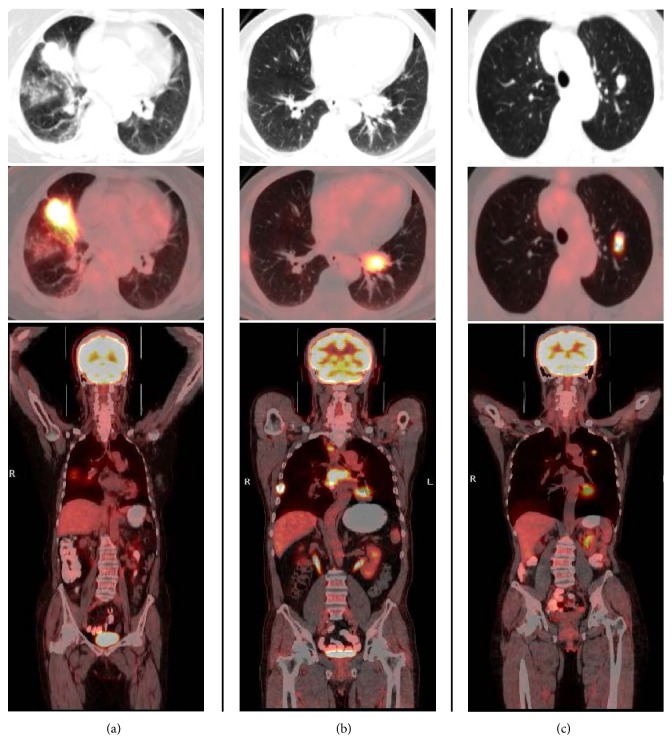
Representative ^18^F-FDG-PET/CT scans. (a) A 60-year-old woman had undergone surgery for rectal carcinoma (stage I) 103 months ago. ^18^F-FDG-PET/CT revealed a solitary pulmonary lesion that was suspected to be malignant (SUV_max_, 13.9). The CEA level was 3.7 *μ*g/L. The patient underwent pulmonary surgery. Pathological examination confirmed pulmonary metastasis. (b) A 65-year-old man had undergone surgery for colon carcinoma (stage II) 50 months ago, followed by adjuvant chemotherapy. PET/CT revealed a solitary pulmonary lesion that was suspected to be malignant (SUV_max_, 7.6), multiple mediastinal lymph metastases, and bone metastasis. The CEA level was 4.6 *μ*g/L. A bronchoscopic biopsy confirmed primary lung adenocarcinoma. (c) A 49-year-old woman had undergone surgery for colon carcinoma (stage II) 36 months ago, followed by adjuvant chemotherapy. PET/CT revealed a solitary pulmonary lesion that was suspected to be malignant (SUV_max_, 8.2). The CEA level was 5.3 *μ*g/L. Pulmonary surgery confirmed granulomatous disease. ^18^F-FDG, ^18^Fluorine-2-fluoro-2-deoxy-d-glucose; PET/CT, positron emission tomography/computed tomography; SUV_max_, maximum standardized uptake value; CEA, carcinoembryonic antigen.
